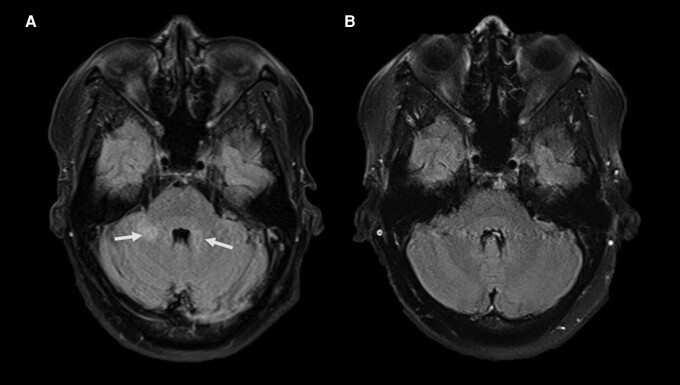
A*, Axial magnetic resonance imaging fluid-attenuated inversion recovery (FLAIR) image, day 7 of illness, demonstrating ill-defined areas of T2-weighted signal hyperintensity in both cerebral peduncles (arrows). *B*, Axial FLAIR image 15 months later demonstrating resolution of the abnormal signal in the cerebral peduncles.
